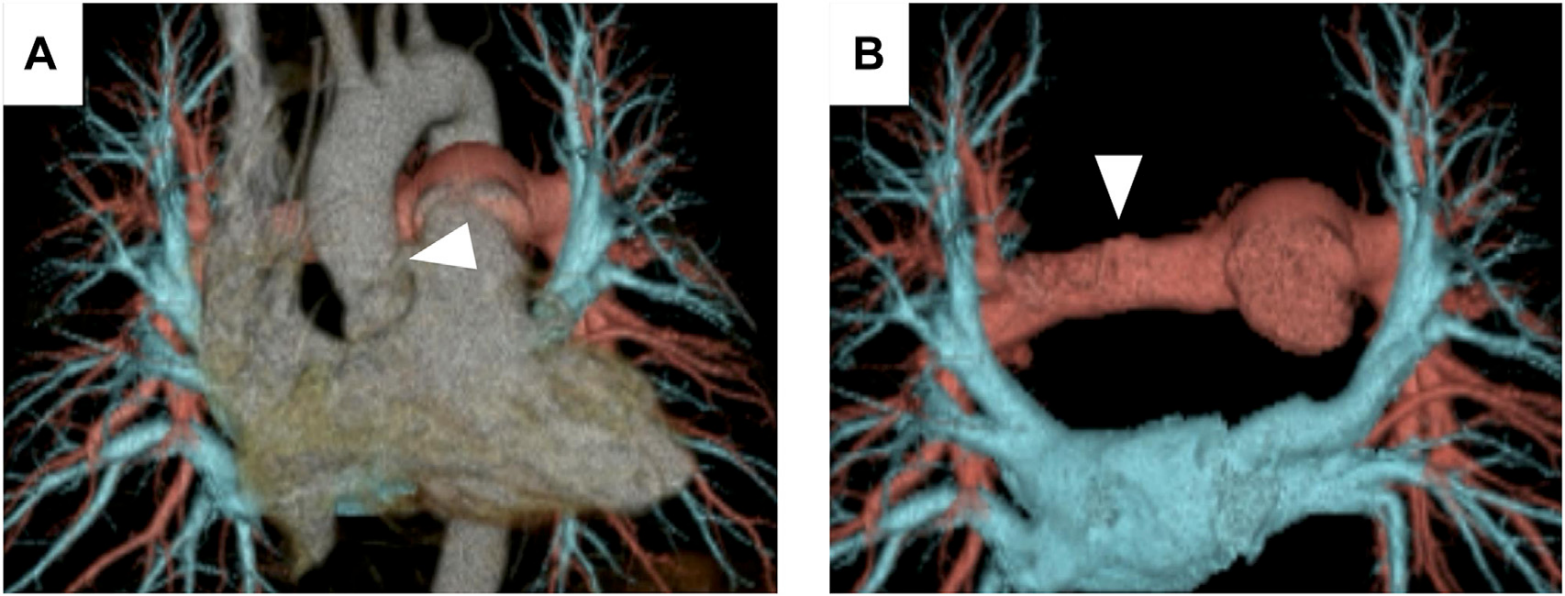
Three-dimensional computed tomography images obtained on postoperative day 5. (A) Reconstructed ascending aorta shows good patency (arrowhead). (B) Reconstructed right pulmonary artery shows good patency (arrowhead).
